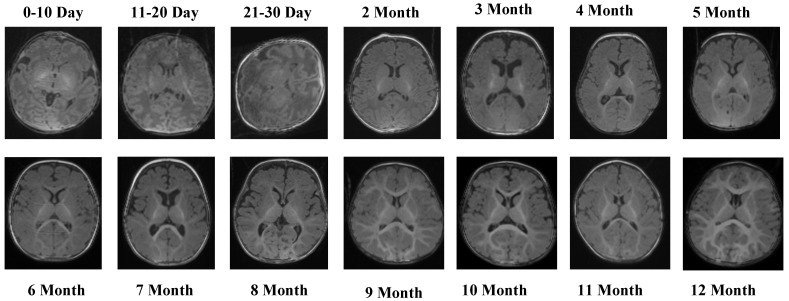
MRI slices from the T1 Female sequence.
